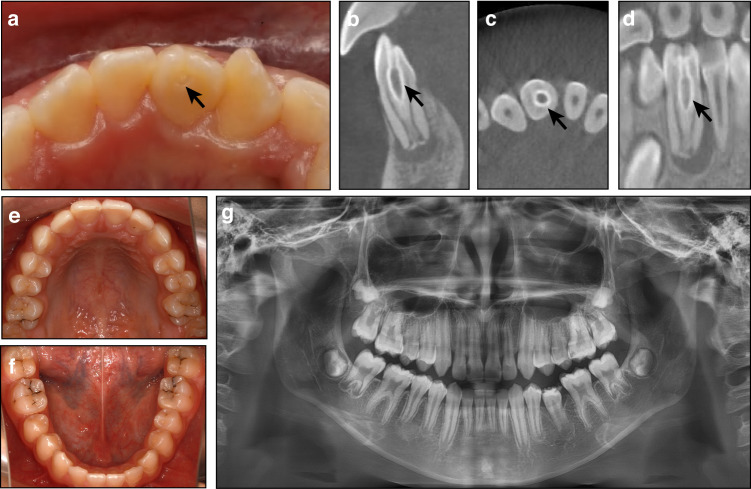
Tooth morphological anomalies. a–d Dens invaginatus. (a) The crown of tooth 41 displays a palatal pit (black arrow). b–d Cone-beam computed tomography reveals a sac-like radiolucency surrounded by a high-density radiopaque border (black arrows). e–g Taurodontism in a 13-year-old male. e, f The external morphology of the teeth appears normal. g The radiograph shows apically positioned pulp chamber floors in the molars
